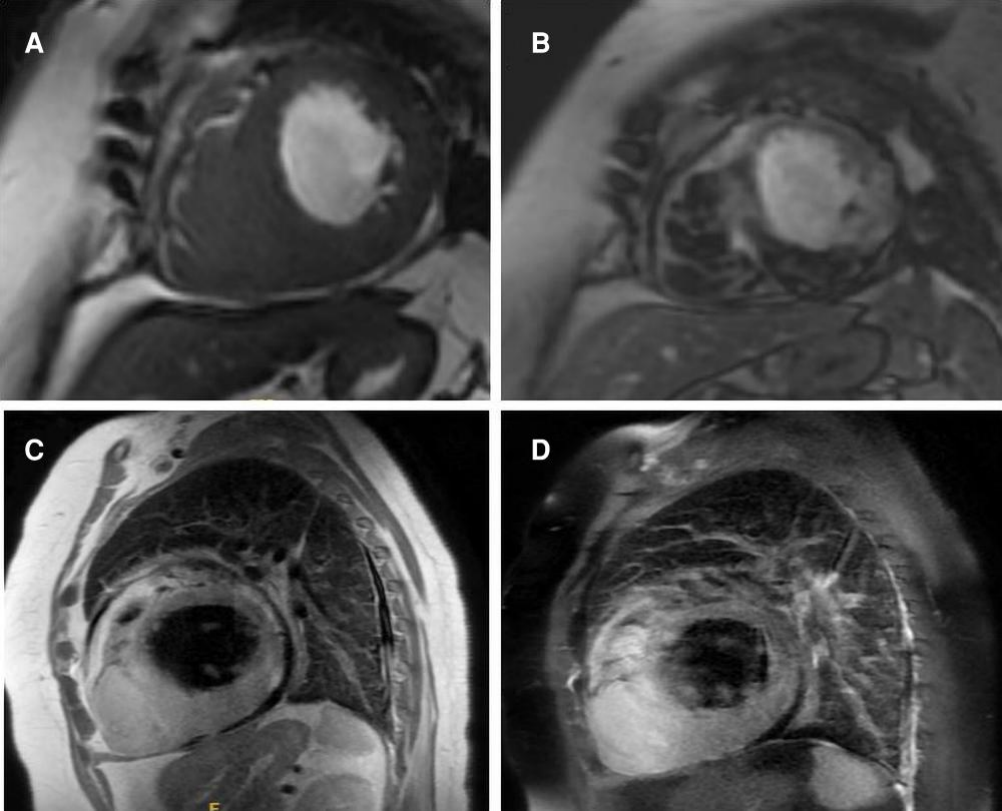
Cardiac magnetic resonance imaging revealed a large infiltrative intracardiac mass, primarily involving the right ventricular apex and infiltrating into the distal interventricular septum and the inferior wall of the left ventricle, as well as part of the adjacent pericardium (*A*). The lesion demonstrates heterogeneous, intense enhancement on LGE sequences, reflecting necrosis, fibrosis, and viable tumour tissue (*B*). On T2-weighted HASTE sequences, the mass appears hyperintense, indicating high water content and cellularity, consistent with a malignant tumour (*C*). On T2-weighted imaging with fat saturation, the mass is isointense to mildly hyperintense relative to myocardium, with suppression of adjacent fat, facilitating clear delineation of the lesion (*D*).
